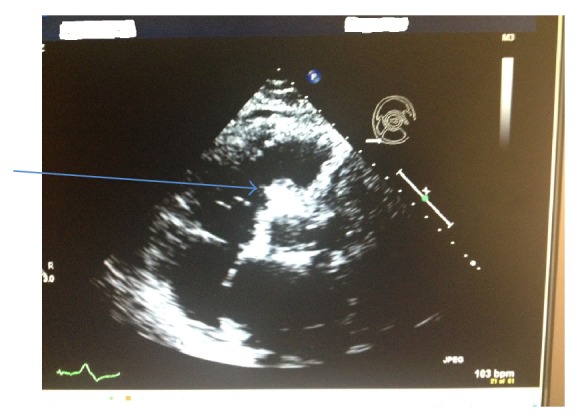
2D echocardiogram shows the large thrombosed sinus of Valsalva aneurysm (blue arrow). Report: Echocardiogram was done showing no change in ejection fraction from previous studies (55%) with left ventricle concentric hypertrophy in addition to the prosthetic aortic valve, dilated left atrium, a very large and thrombosed sinus of Valsalva aneurysm, mildly dilated inferior vena cava, a pacemaker lead in the right ventricle, and a catheter/pacemaker lead seen in the RA cavity. It was noted that the right ventricle systolic pressure was elevated at 40–50 mmHg.
